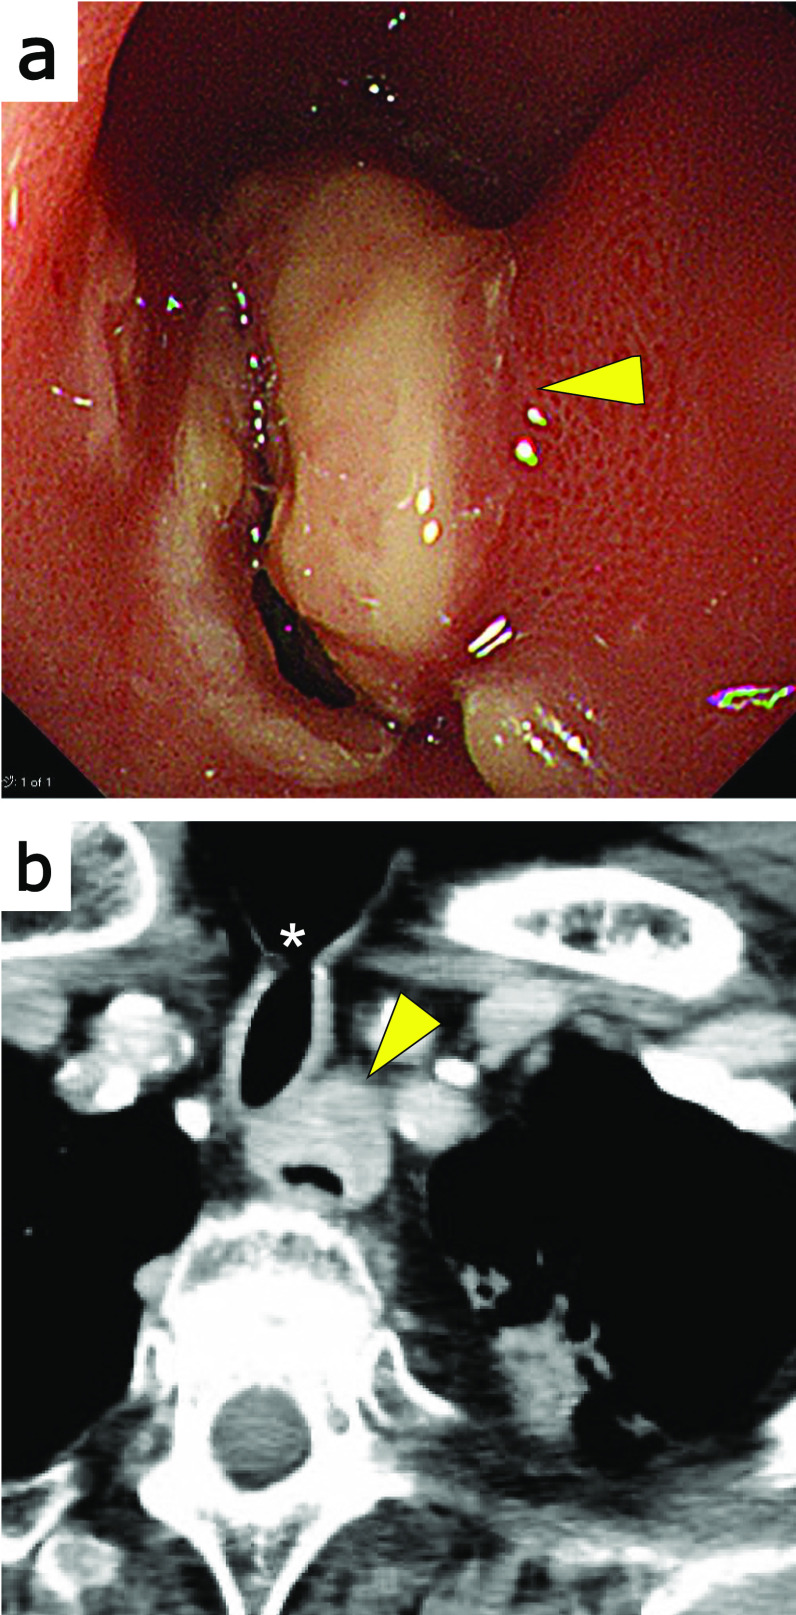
Preoperative findings. a Upper gastrointestinal endoscopy observed a tumor just below the esophago-jejunal anastomosis (yellow arrowhead). b Contrast-enhanced CT image showed the tumor at the esophageal side of the esophago-jejunal anastomosis (yellow arrowhead). Asterisk indicates tracheostomy
